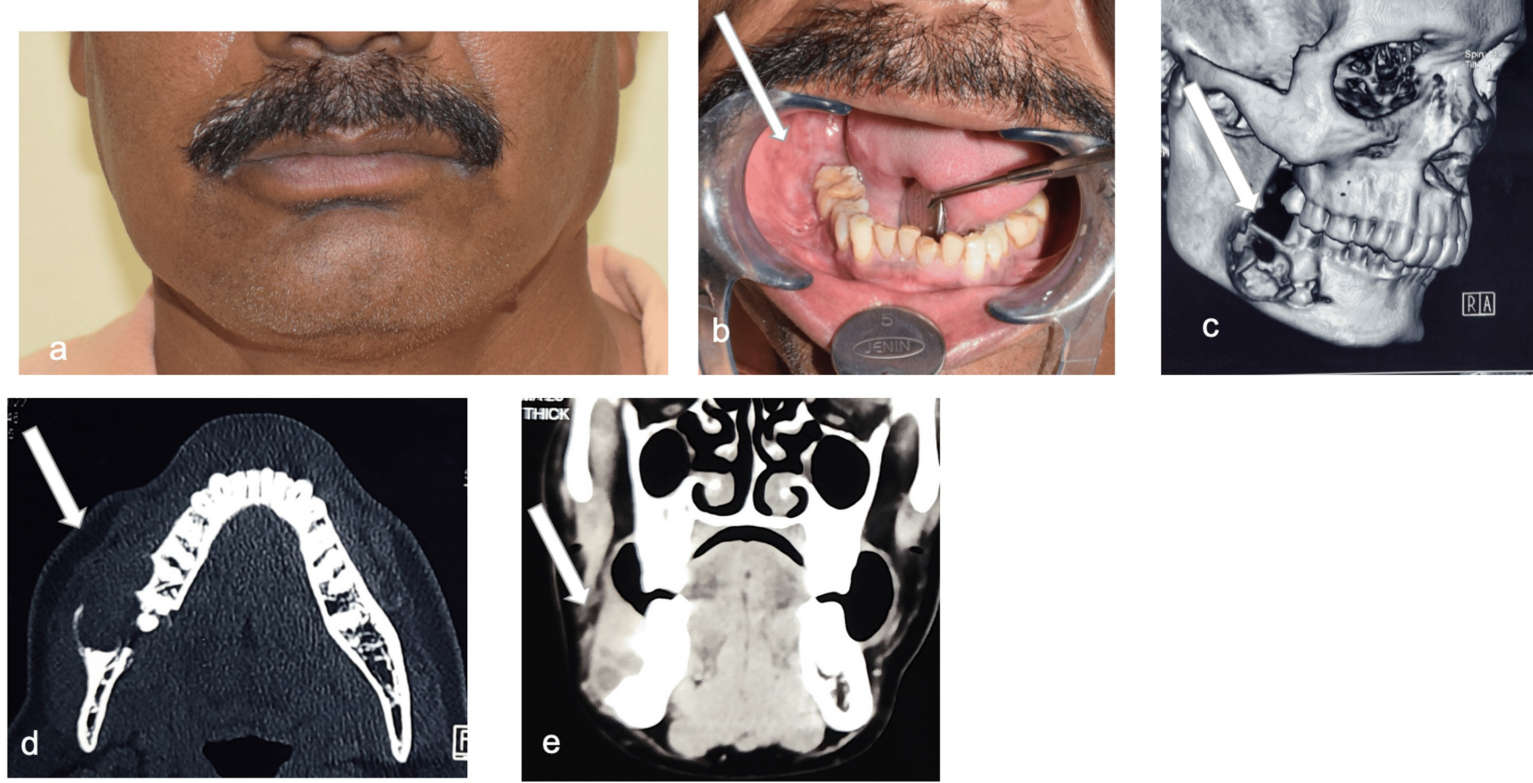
a: Extraoral image. b: Intraoral image. c: CECT showing an osteolytic lesion. d. Axial section. e. Coronal section. CECT: Contrast-enhanced computed tomography
